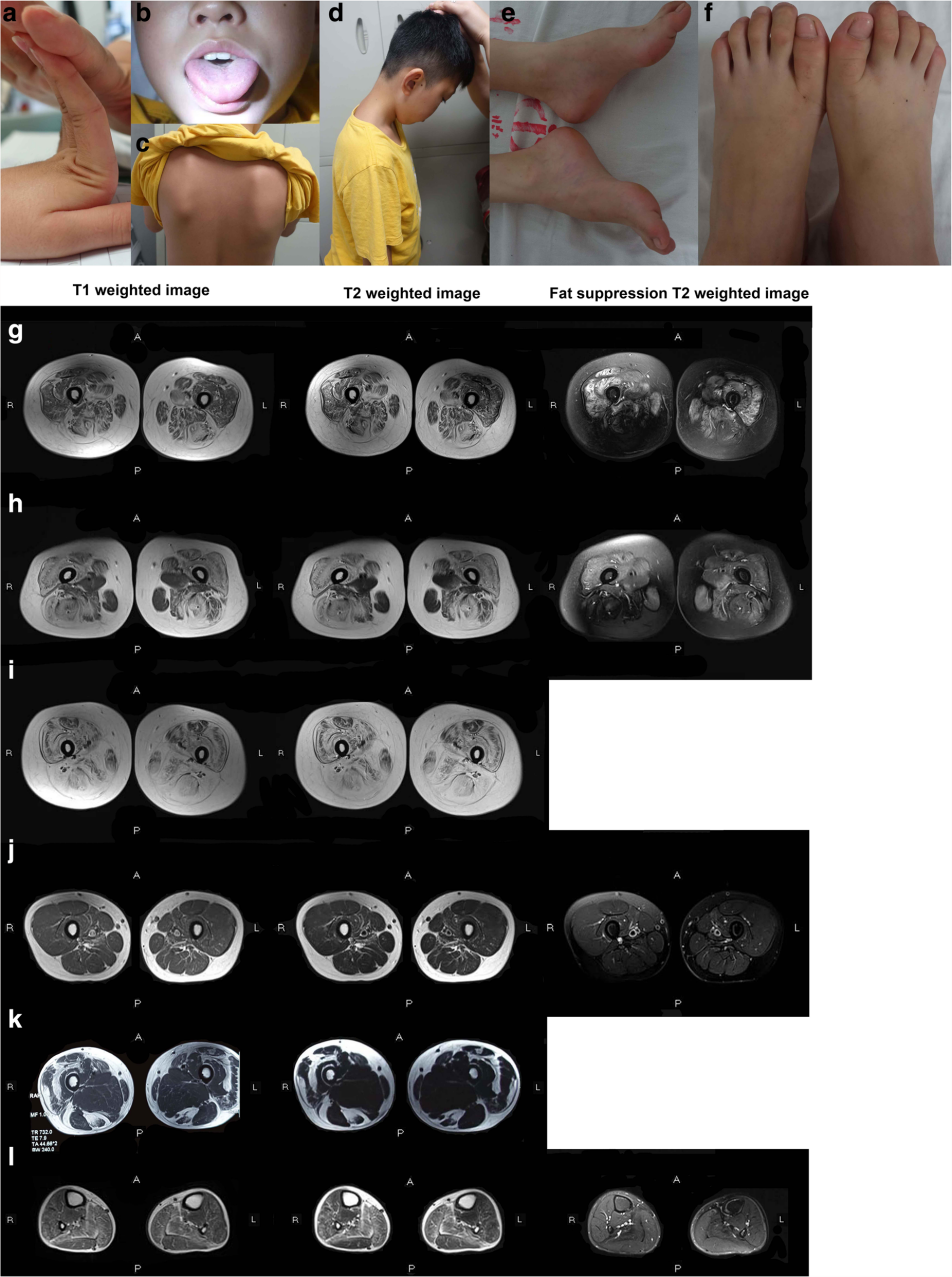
Clinical and magnetic resonance imaging (MRI) results obtained for all patients included in the study. (a) Hypermobility of the metacarpophalangeal joints observed in proband 11. (b–e) Physical characteristics of proband 12. (b) Tongue hypertrophy. (c) Winged shoulder. (d) Neck contracture. (e) Ankle contracture and cavus feet. (f) Morton’s toe. (g–l) Muscle MRI results obtained for probands 3, 5, 9, 11, and 12. T1-weighted image, T2-weighted image, and fat-suppression T2-weighted image (left to right). MRI results of both thighs obtained for proband 3 (g), proband 5 (h), proband 9 (i), proband 11 (j), and proband 12 (k). (l) MRI results of both calves obtained for proband 12
